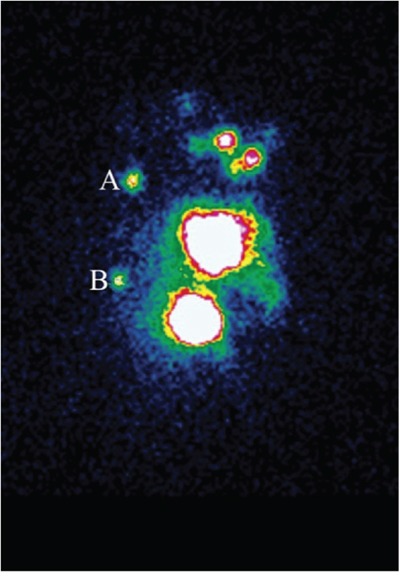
Preoperation lymphoscintigram with radiotracer Hotspots are evidently shown in both the second intercostal space A. and the fourth intercostal space B. in patient with left-sided breast cancer.
